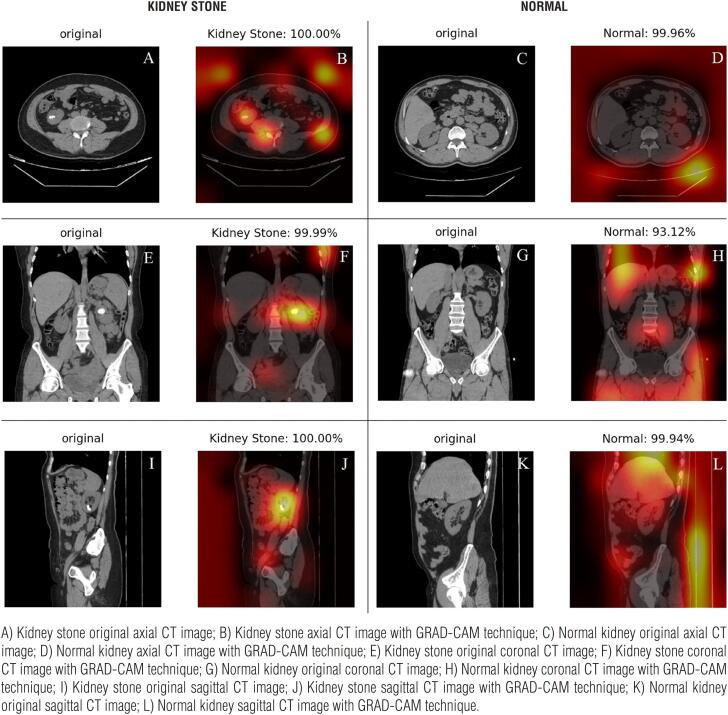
Axial, sagittal, and coronal CT images of the patients bearing kidney stones greater than 2 cm in size and without kidney stones, demonstrated with the Grad-CAM technique. Percentages refer to the estimates of the AI model.
